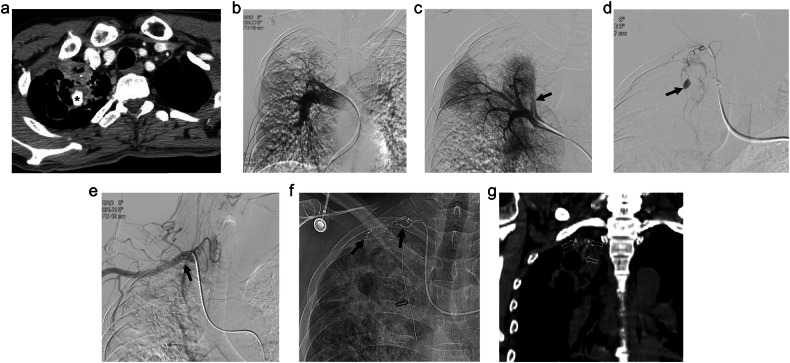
A 47-year-old male was admitted with cough and massive hemoptysis. The patient had a history of pulmonary tuberculosis. a The CT scan reveals the presence of a pulmonary artery pseudoaneurysm (PAP) (*) in the right upper lobe of the lung. Surrounding tuberculous cavity and consolidation due to tuberculous pneumonia can also be observed. b Pulmonary arteriography was performed using a pigtail catheter; however, the PAP could not be visualized. c Upon selective arteriography, the suspected pulmonary arterial branch supplying the PAP was not opacified owing to competitive systemic arterial flow (arrow). d Upon suspecting that the PAP received supply from the systemic artery, bronchial and non-bronchial systemic arteriography were performed to evaluate potential collateral arteries. The PAP was visualized via systemic-to-pulmonary artery shunt on the selective arteriography of the branch of the subclavian artery (arrow). e, f Embolization was performed via systemic-to-pulmonary shunt using a mixture of N-butyl cyanoacrylate (NBCA) and lipiodol (ratio 1:3). Embolic material casted in the main feeding systemic arterial branches of the PAP (arrows). Upon performing postembolic subclavian arteriography, the PAP was not visualized. Microcatheter was located in the internal mammary artery for the additional bronchial/non-bronchial systemic artery embolization (BAE/SAE) on the fluoroscopic image (open arrow). g Precontrast CT scan shows embolic material casted in the peripheral pulmonary artery around the PAP (open arrows), which was barely visualized on the fluoroscopic images. After embolization, the patient underwent additional BAE/SAE, and massive hemoptysis stopped
